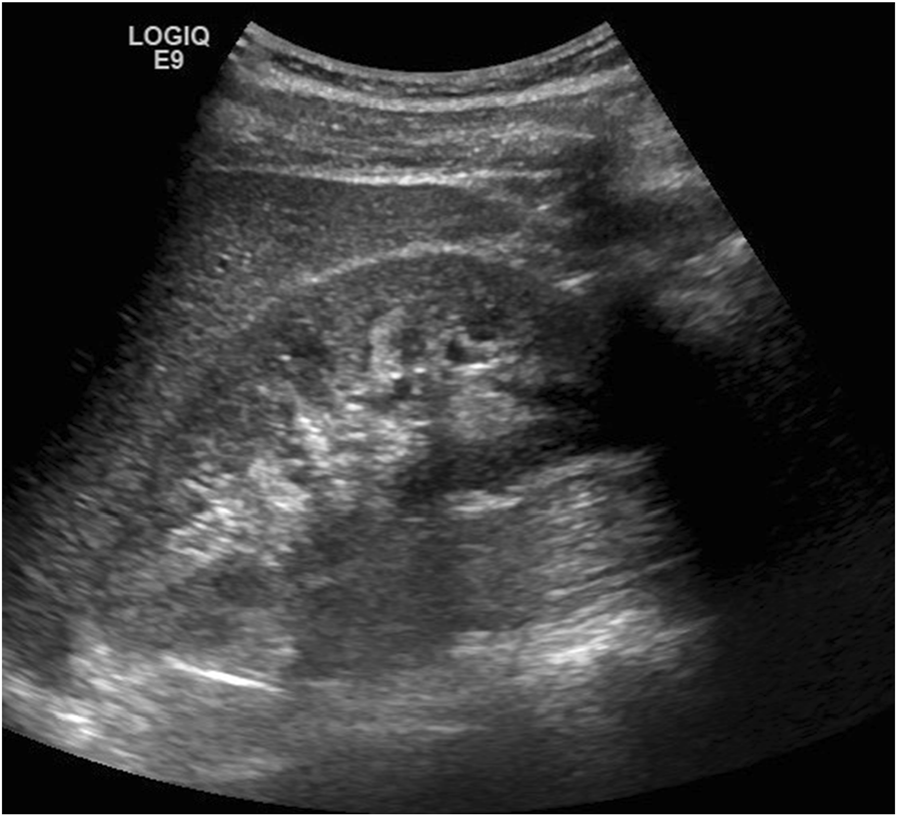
Ultrasound localisation of kidney in live model
